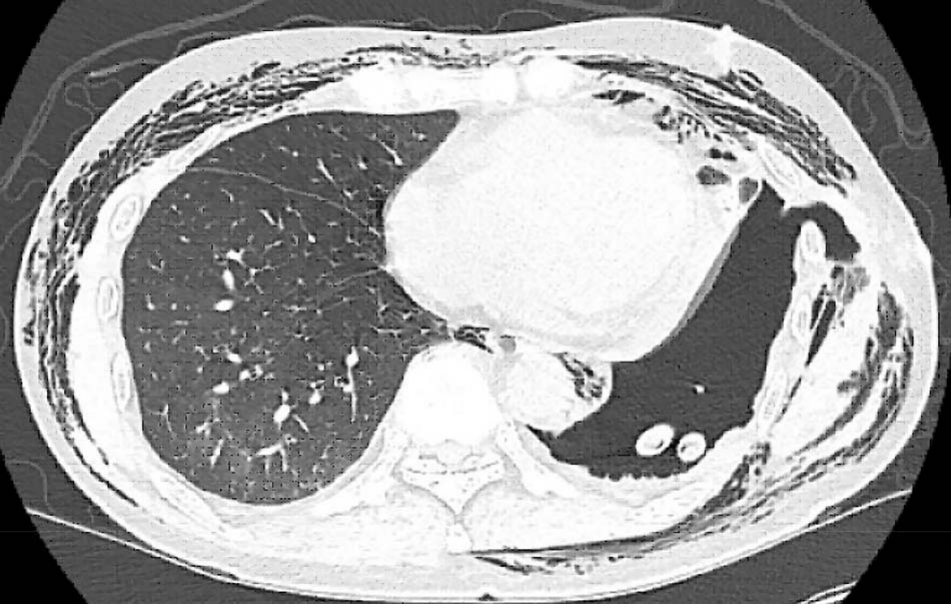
POD13 CT scan demonstrating a BPF between the fifth and sixth ribs communicating with a pneumothorax and subcutaneous emphysema.
